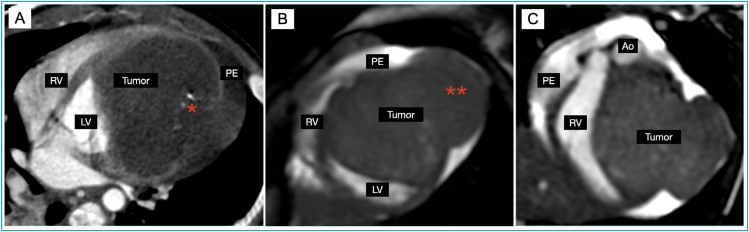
Cardiac angiotomography (A) and cardiac magnetic resonance imaging (B and C), in the 4-chamber (A and B) and 2-chamber short axis (C) views, demonstrating a large expansive lesion in the apicobasal anterolateral wall of the left ventricle (LV), with reduction of left ventricular volume, small foci of central calcifications (*in A), extracardiac extension (in C) and pericardial effusion (PE in A-C).
